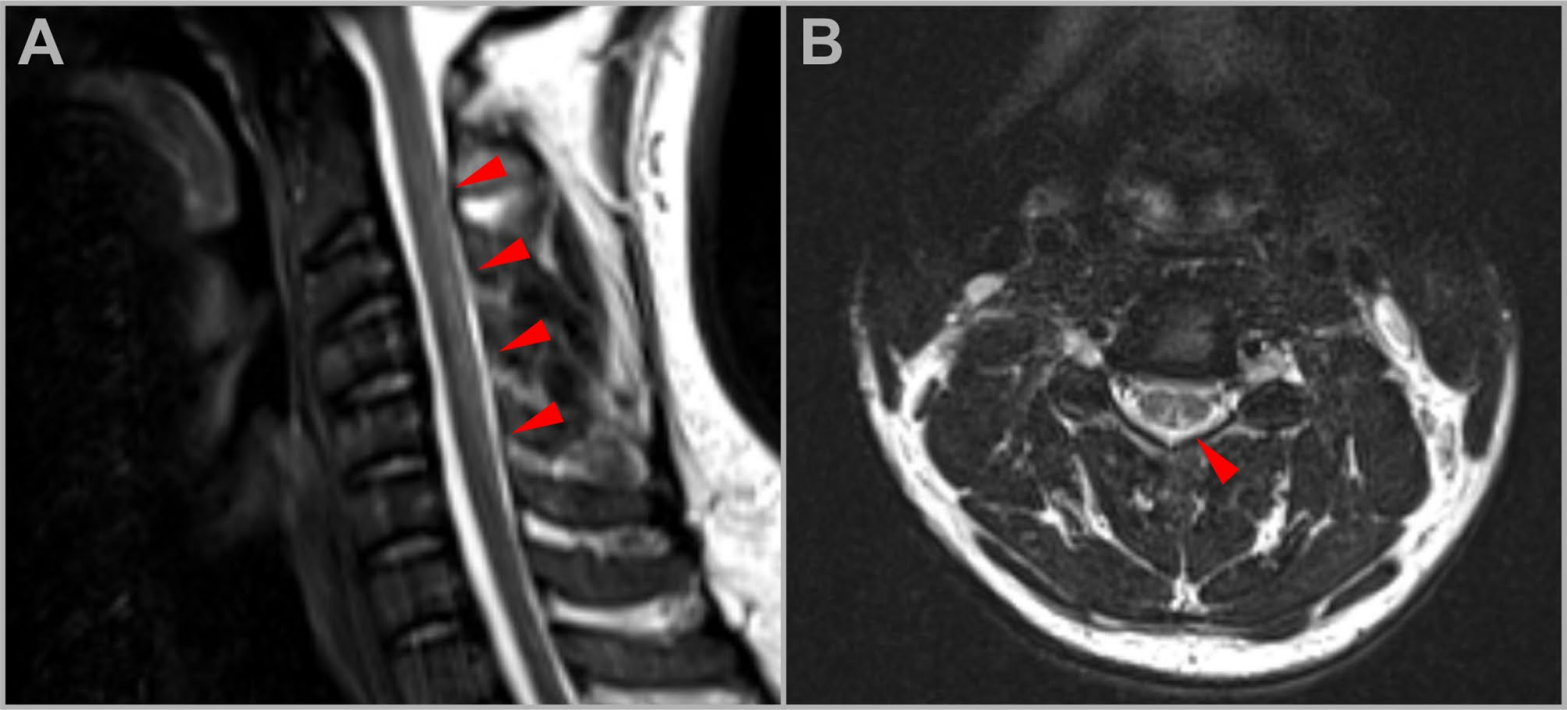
MRI findings in N₂O-associated myelopathy. Sagittal (A) and axial (B) T2-weighted MRI images of the cervical spine. (A) Sagittal T2-weighted image demonstrates longitudinally extensive hyperintense signal abnormalities within the dorsal columns of the cervical spinal cord (red arrowheads). (B) Axial T2-weighted image shows a characteristic V-shaped hyperintensity involving the dorsal aspect of the spinal cord (red arrowhead), consistent with selective involvement of the posterior columns
